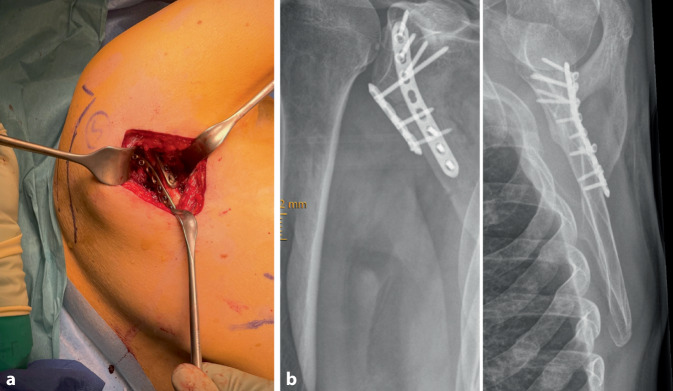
a The 2.7 mm plate should be precontoured keeping the glenopolar angle (30–40°) in mind. The plate is positioned on the dorsal aspect, along the lateral border of the scapula. Preliminary fixation with K‑wires is advisable. b It is important in this phase to obtain adequate intraoperative radiographs with a free projection of the joint glenohumeral joint to exclude intra-articular positioning of the most cranial K‑wire. The direction of the cranial K‑wire may be used as a reference when placing the screws in the cranial part (near the glenohumeral joint) of the plate. At the end of the procedure multidirectional intra-operative radiographs should be obtained
